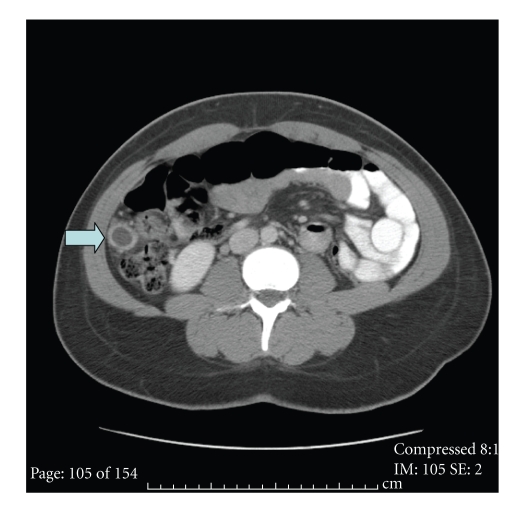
Computed tomography (CT) imaging of the abdomen revealing a 2.2 cm complex cystic structure 2 cm inferior to the inferior hepatic border which was suspicious for an ectopic abdominal gestational sac.
